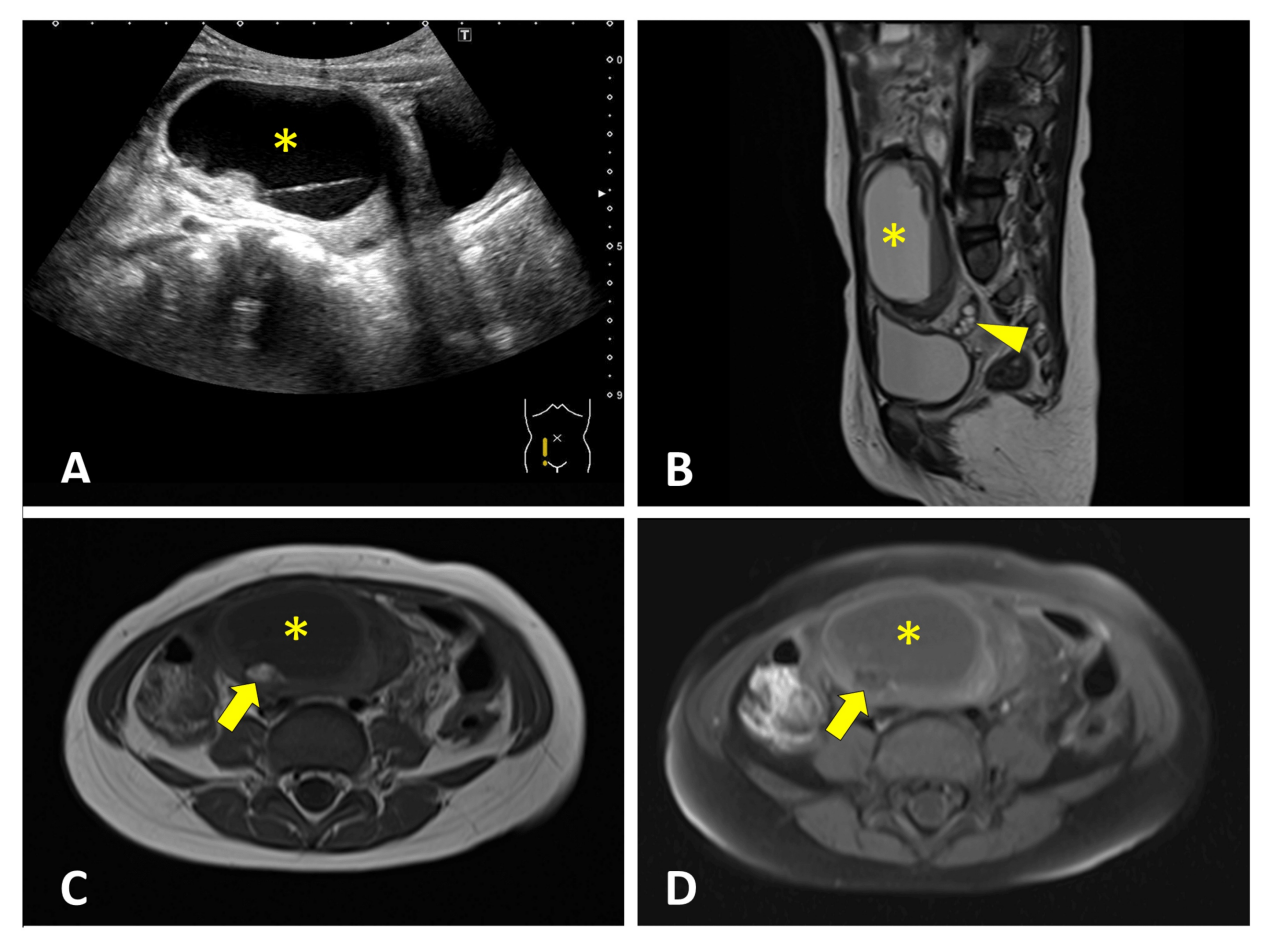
Double-Bladder Sign on RADUS With Corresponding MRI Findings Longitudinal RADUS (A) demonstrated a cystic mass (*) producing the double-bladder sign. Sagittal T2-weighted MRI (B) demonstrated a corresponding large cystic lesion (*), with the normal right ovary located posterior to the lesion (arrowhead). Axial T1-weighted and T1 fat-suppressed images (C, D) suggested the presence of intralesional fat (arrow). RADUS: radiology-performed ultrasound
